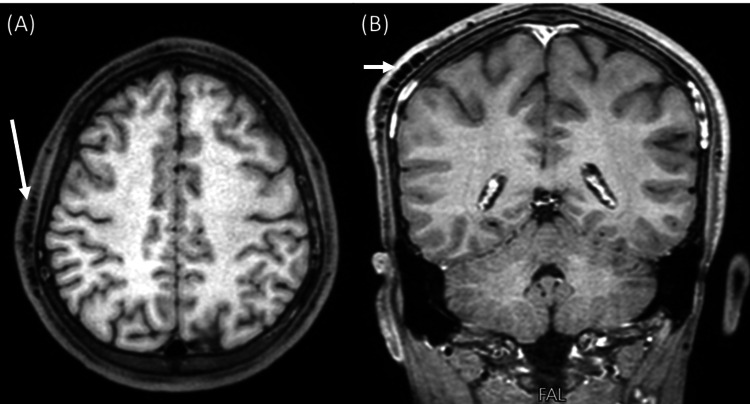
Axial (A) and coronal (B) T1-weighted MRI show tortuous dilated signal void vessels in the scalp of the right parietal region
